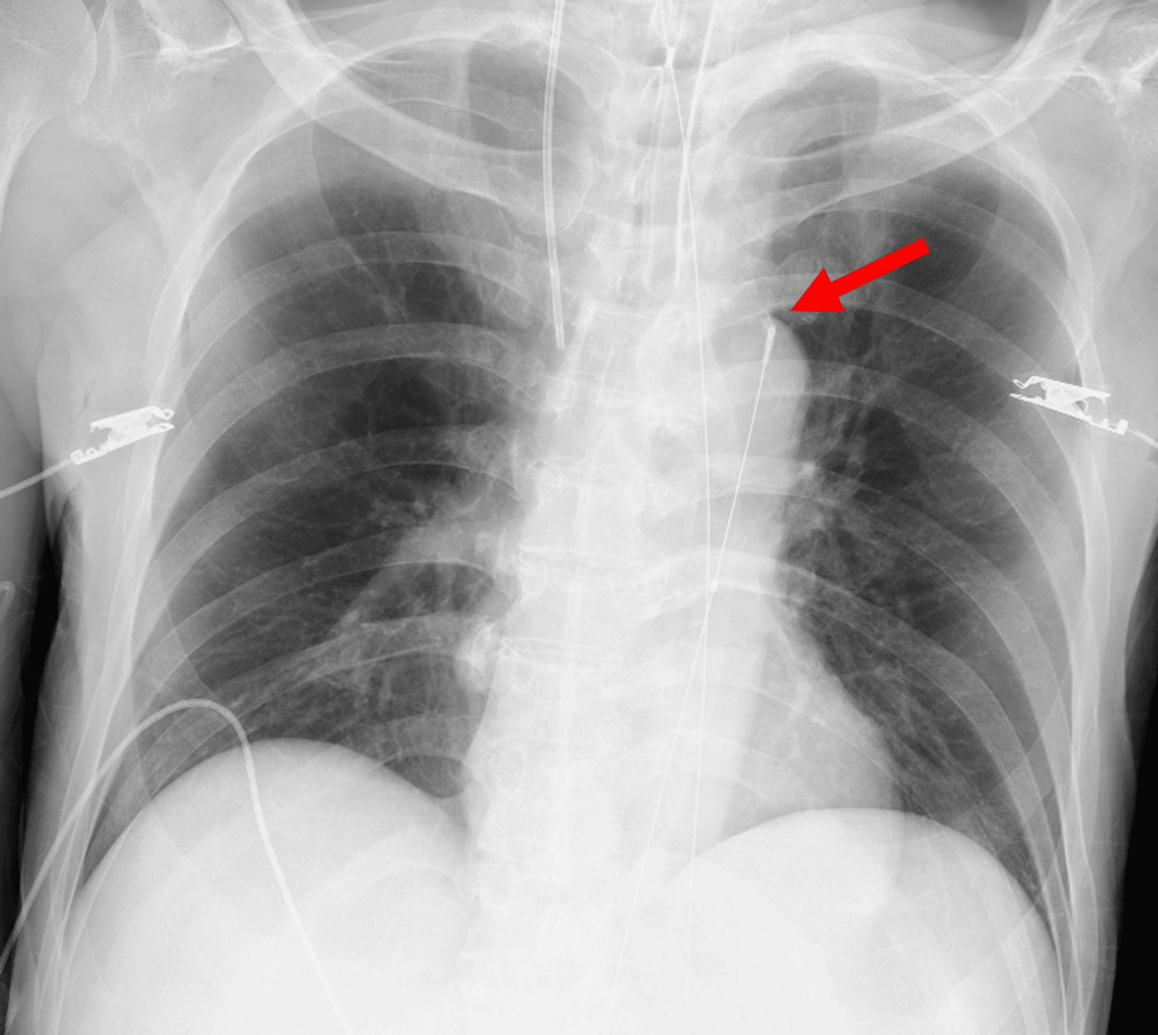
Chest X-ray demonstrating the REBOA catheter (Case 2) The red arrow indicates the tip of the REBOA catheter, which is appropriately positioned. REBOA: Resuscitative endovascular balloon occlusion of the aorta
